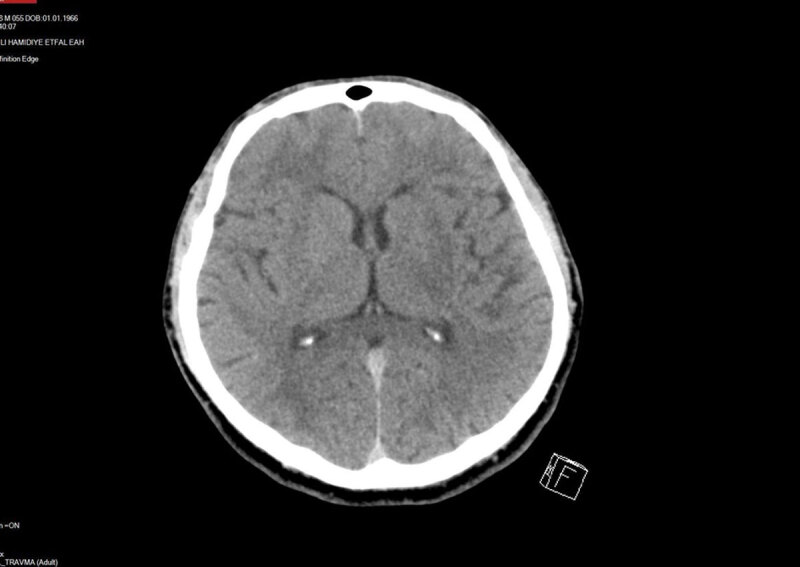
Unenhanced axial CT scan shows bilateral symmetric hypodensities along both lentiform nuclei and cerebral cortical white matter.
